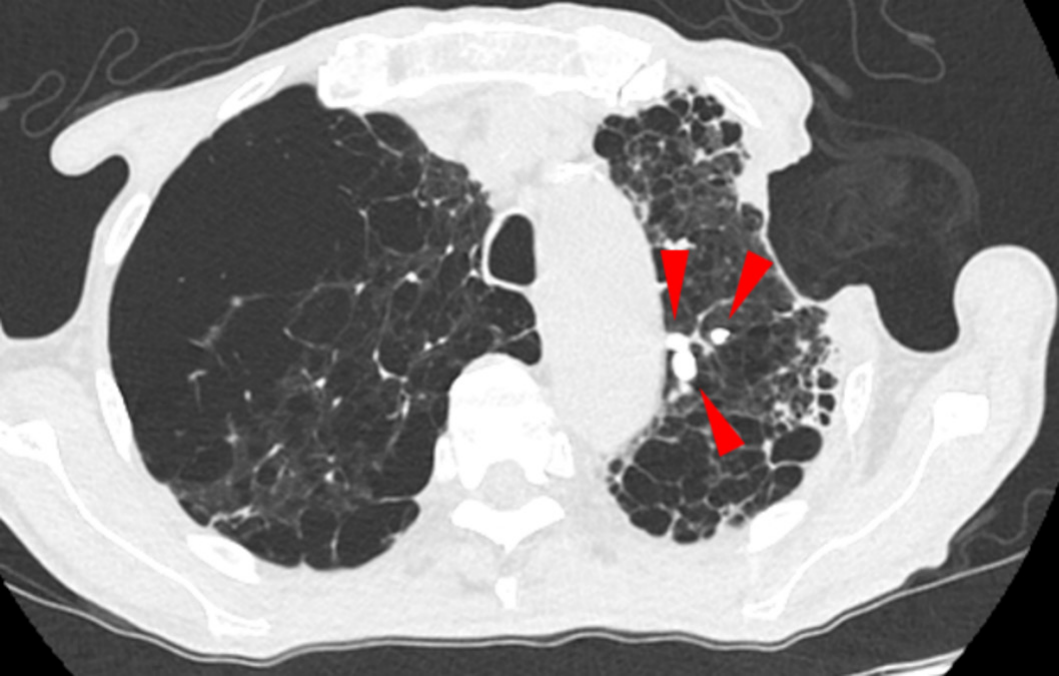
Computed tomography after endobronchial Watanabe spigot (EWS) placement. The red arrowheads indicate the EWS plugs.
